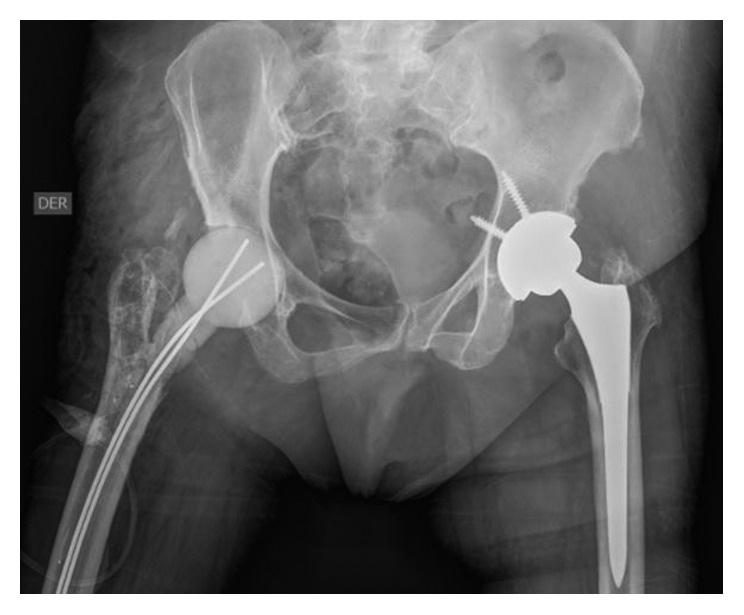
Postoperative radiograph after fistula resection, complete prosthesis removal, surgical lavage and debridement, tissue cultures, and vancomycin cement spacer were performed.
